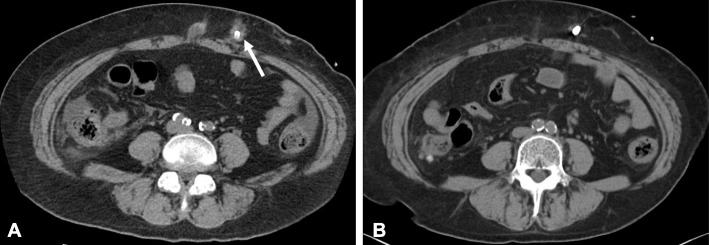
Abdominal computed tomography images in this patient. Images were obtained before subcutaneous pathway diversion (a) and at admission for peritonitis (b). Subcutaneous abscess around the catheter is indicated by arrow in a
